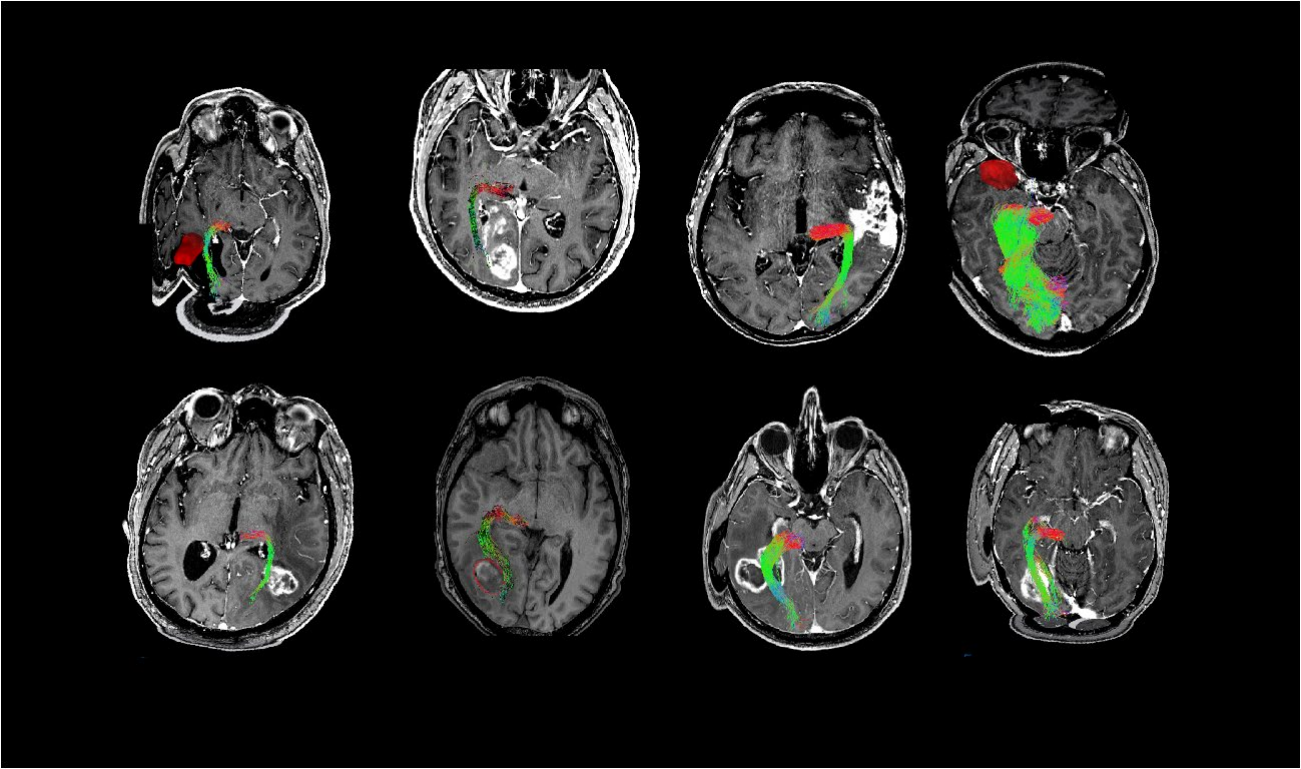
Overview of lesions and FT results (in numerical order). All MRI T1-weighted sequences, oblique view. FT all according to QBI. For patient 4, overlay of DTI-FT (pink) and QBI-FT (purple). MRI = magnetic resonance imaging; FT = fiber tractography; QBI = q-ball imaging; DTI = diffusion tensor imaging
